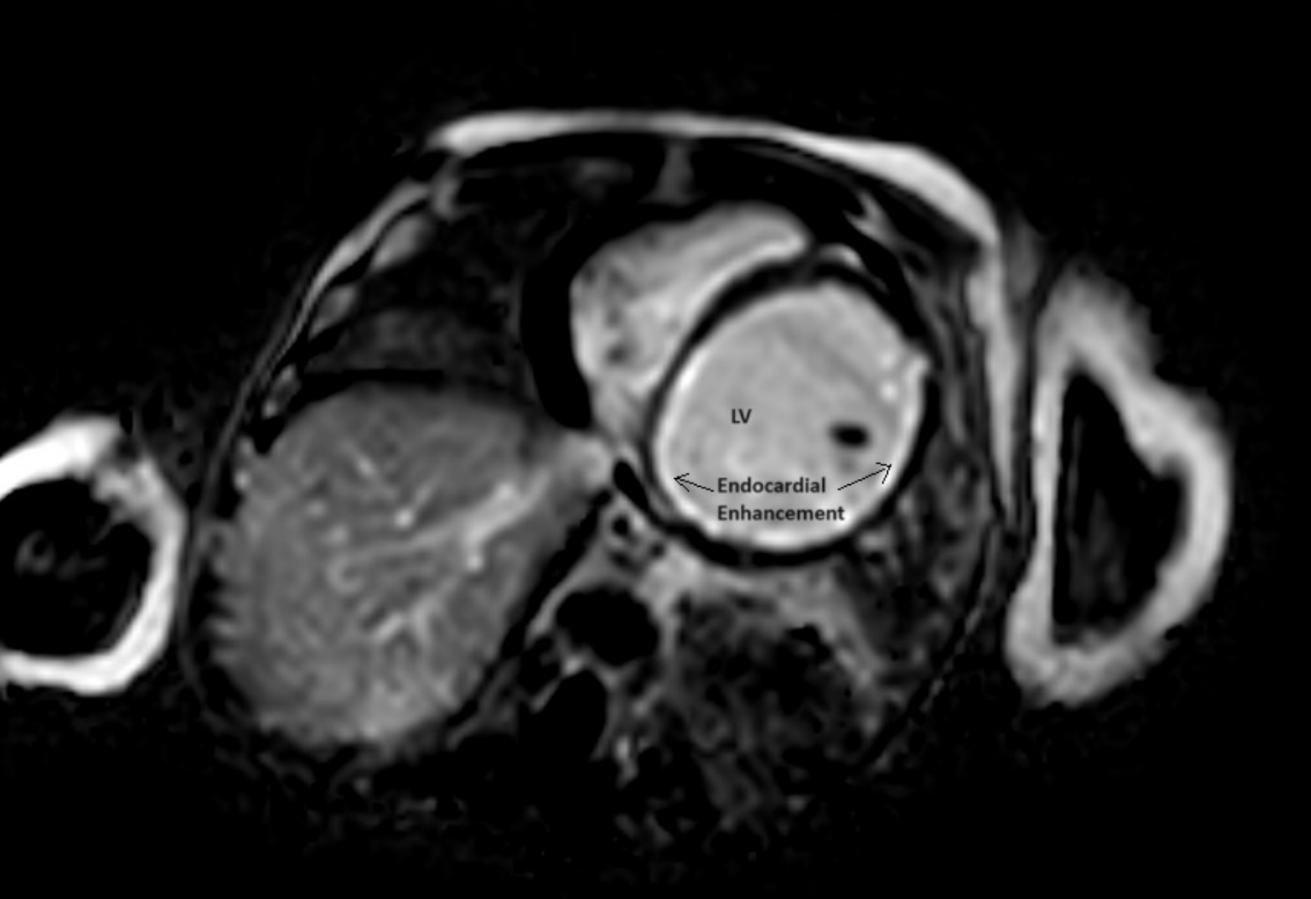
Cardiac MRI scan - Diffuse LV late gadolinium enhancement
